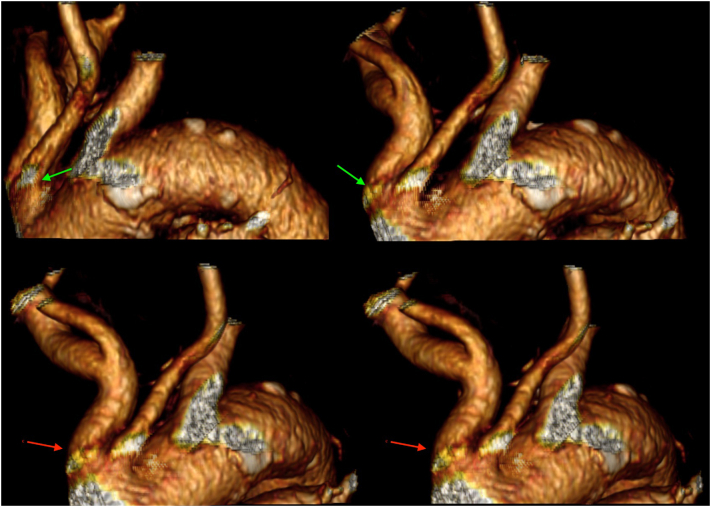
Preoperative CT Angiography 3-dimensional volume rendering showing aortic arch and left common Artery from different angles. The common origins of brachialcephalic artery and left common carotid are an important anatomical obstacle to the passage with access from femoral or radial access.
